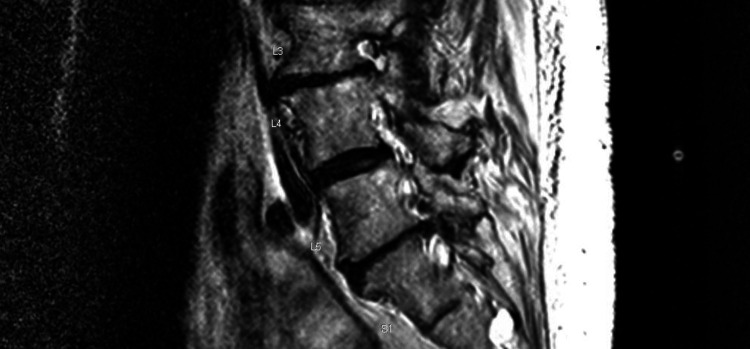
Sagittal MRI with facet arthropathy and L4-L5 anterolisthesis.
